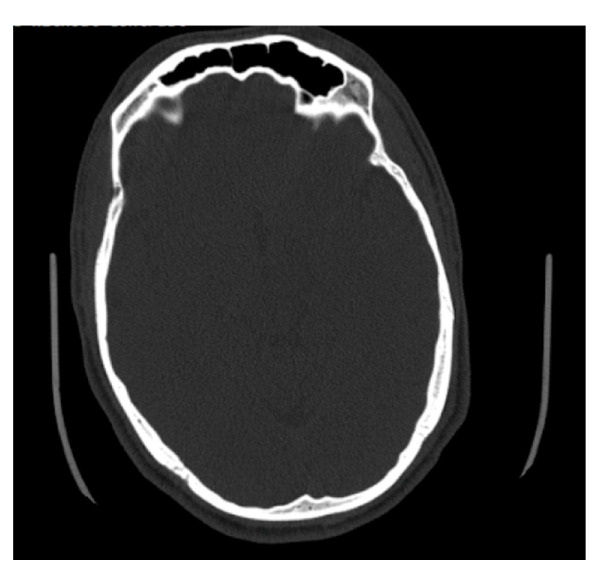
Example of an axial CT image slice useful for measuring frontal sinus depths.
